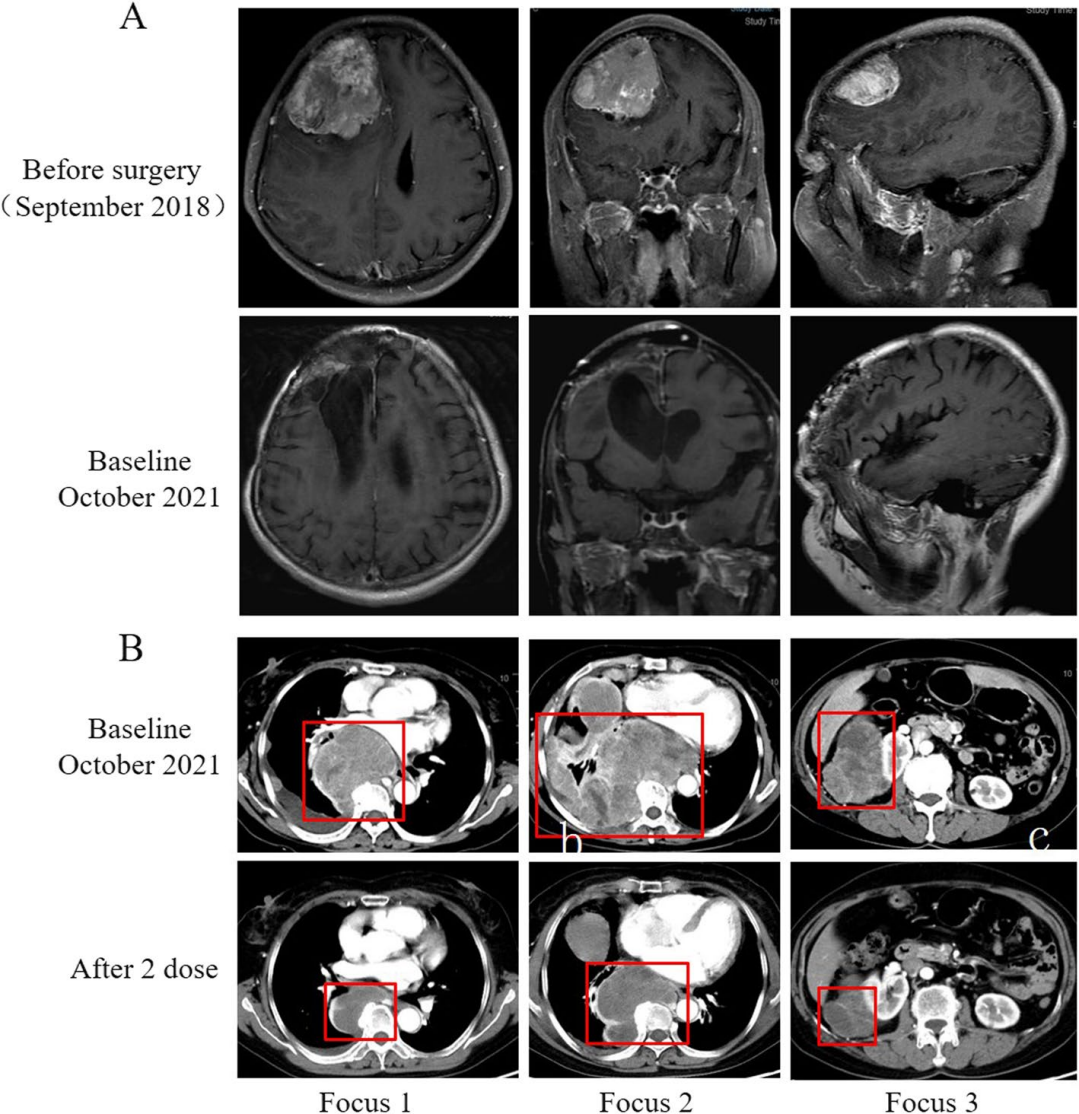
A Magnetic resonance imaging (MRI) of the primary meningioma. MRI scans showed that the primary meningioma was located in the right frontal lobe of the convexity of the brain and could be completely resected. B Computed tomography (CT) revealed significant shrinkage of the tumor after two cycles of treatment compared with the size of the tumor before treatment
